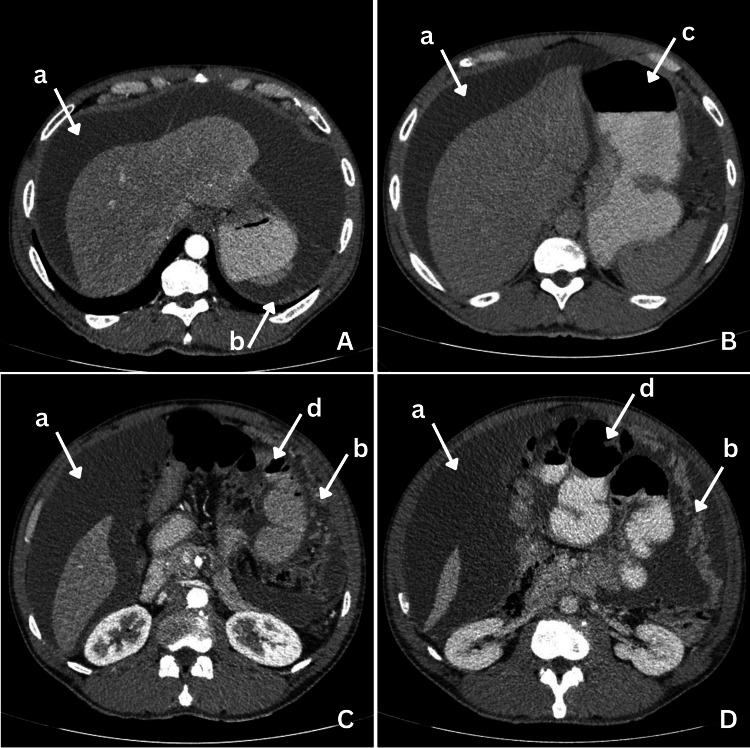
Abdominal CT Scan Abdominal CT scan with oral (A, B, C, and D) and intravenous contrast (A and C). (a) Ascites, (b) images suggestive of carcinomatosis peritoneal, (c) the distension of the gastric chamber and the alteration of the wall, and (d) distended small bowel loops CT: computed tomography
